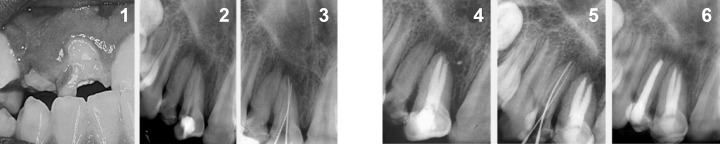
1) Photographic image of teeth #7 and #8, 2) radiographic image of teeth #7 and #8, 3) working length measurement of tooth #8, 4) postoperative radiograph of tooth #8, 5) working length measurement of tooth #7, 6) postoperative radiograph of teeth #7 and #8
